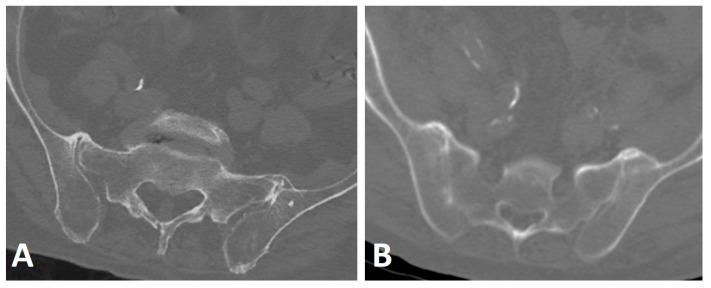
Axial CT images of the SIJs of two patients with DISH. (A) Characteristic extra-articular bridging osteophytes are seen anterior to the SIJs. (B) In addition to the ventral extra-articular osteophytes, clear ankylosis can be observed within the right and left SIJs.
